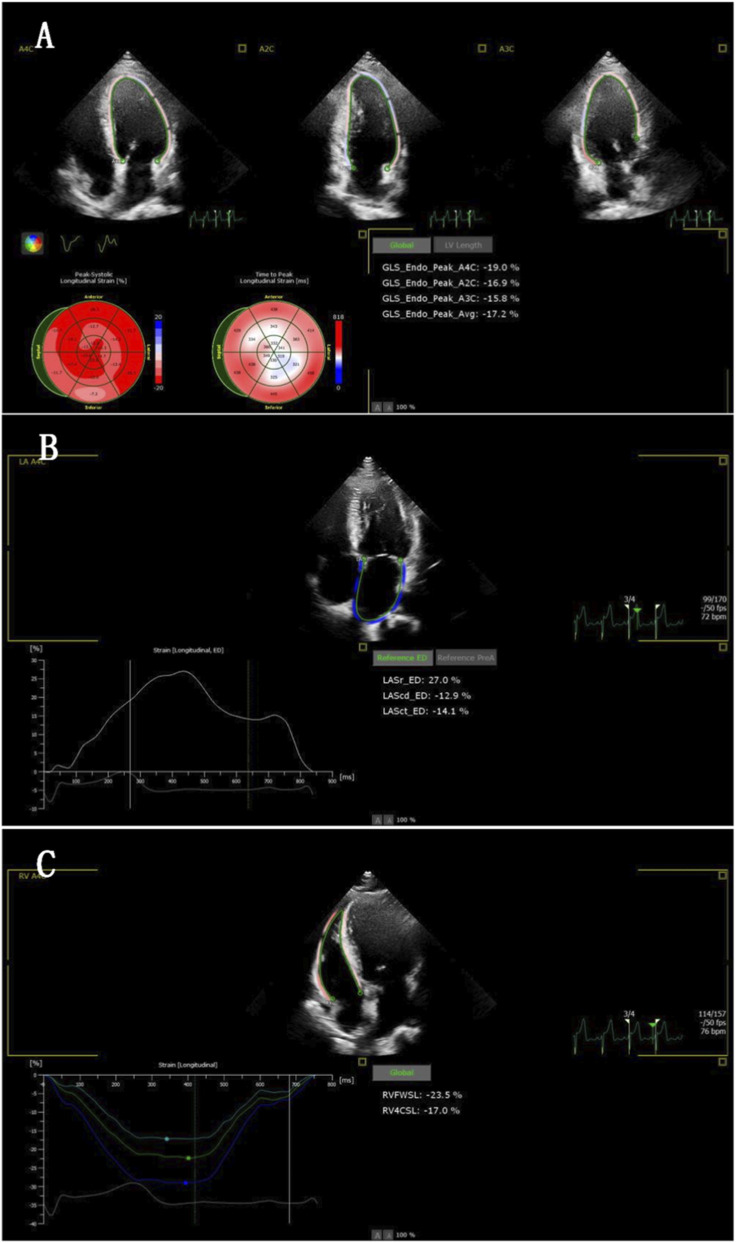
Strain analysis by 2D-STE. (A) Measurement of global longitudinal strain (GLS) in the apical 4-chamber, 2-chamber, and 3-chamber views. (B) Measurement of left atrial strain (LAS), including reservoir strain (LASr), conduit strain (LAScd), and contractile strain (LASct), in the apical 4-chamber view. (C) Measurement of right ventricular free wall longitudinal strain (RVFWSL) in the 4-chamber view.
